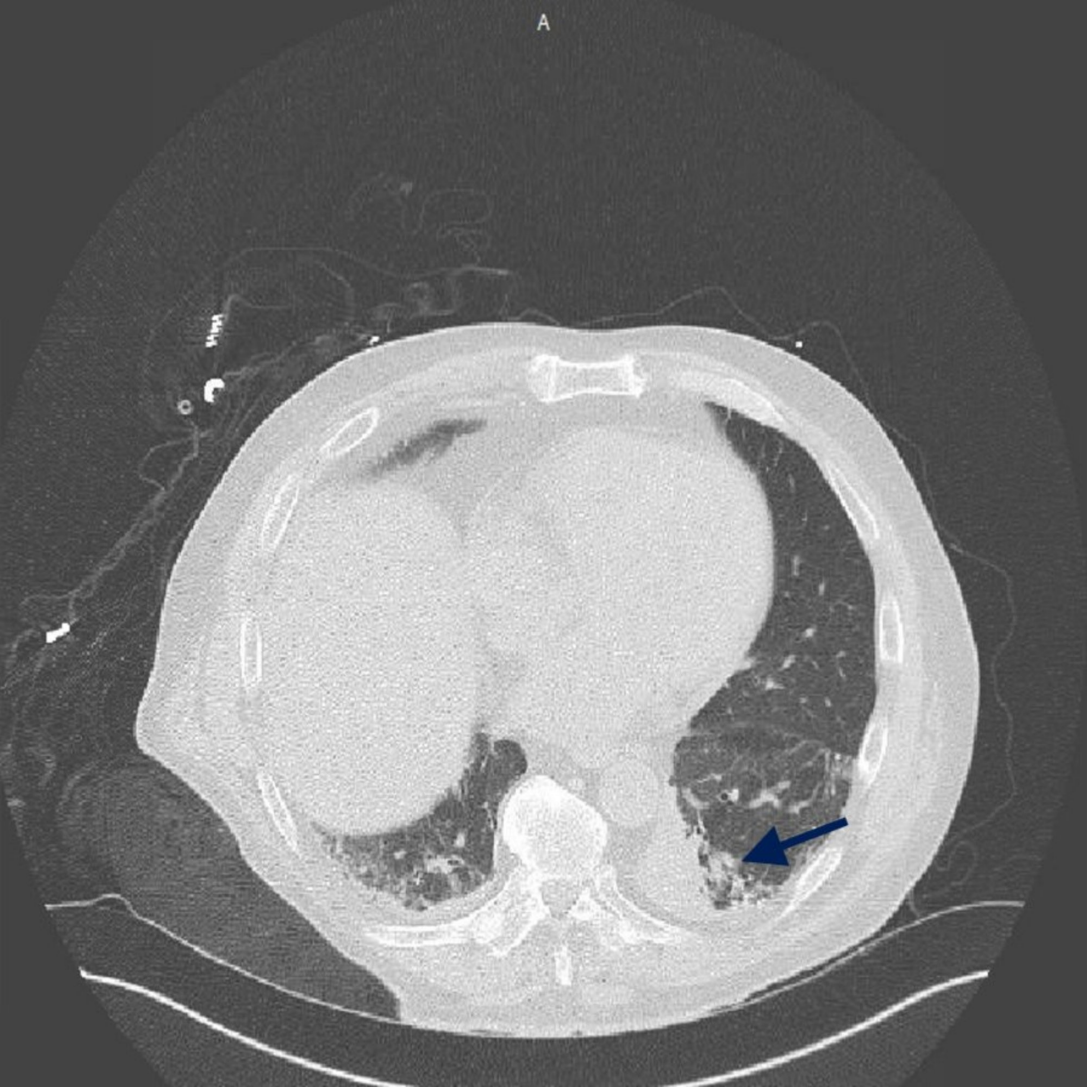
Computed tomography scan of the chest showing consolidation within medial portion of the left lower lobe (blue arrow).
